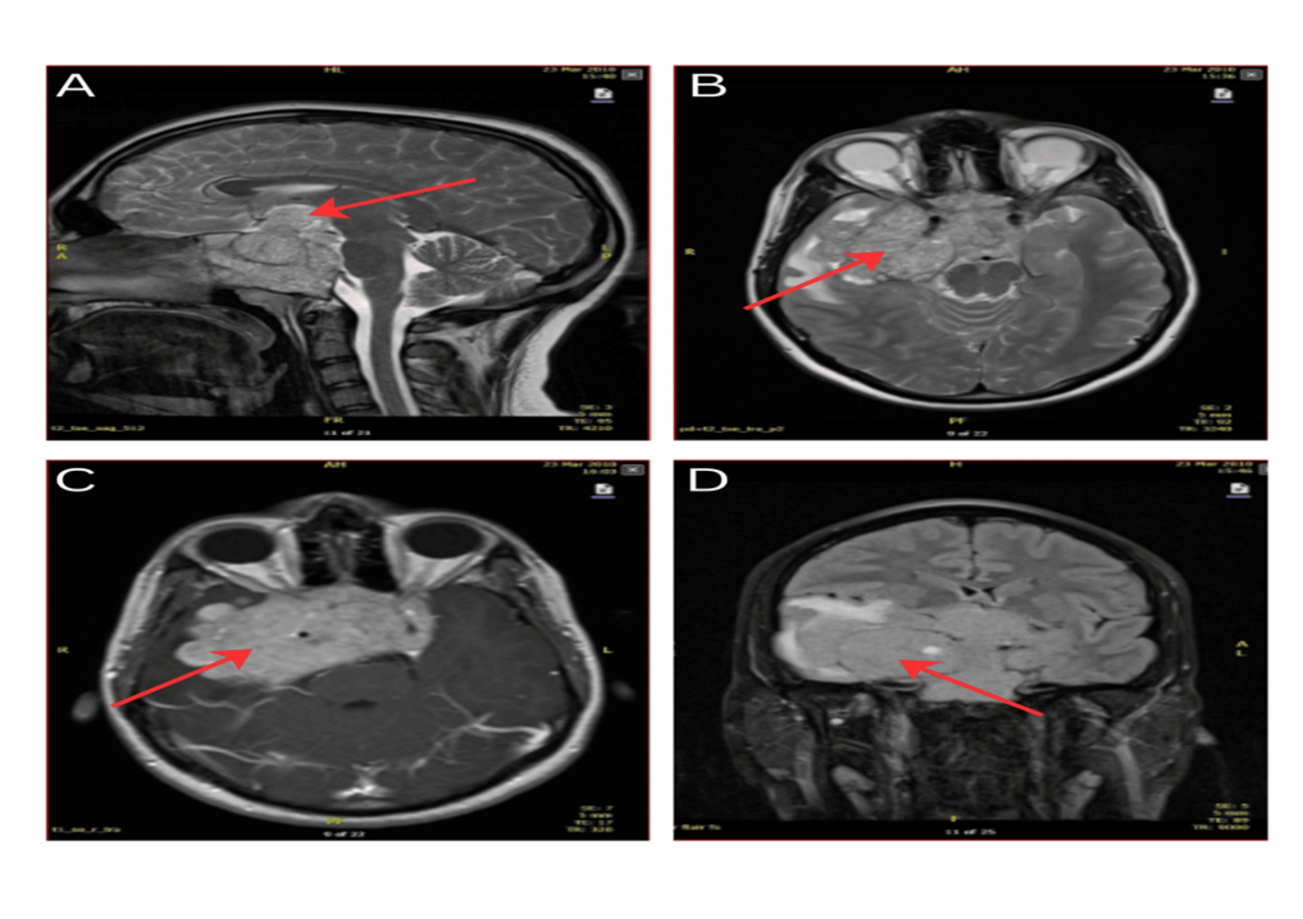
2010 MRI brain exhibiting pituitary mass (indicated by red arrows) with extension into right temporal lobe. A: Sagittal view; B and C: transverse view; and D: coronal view.
